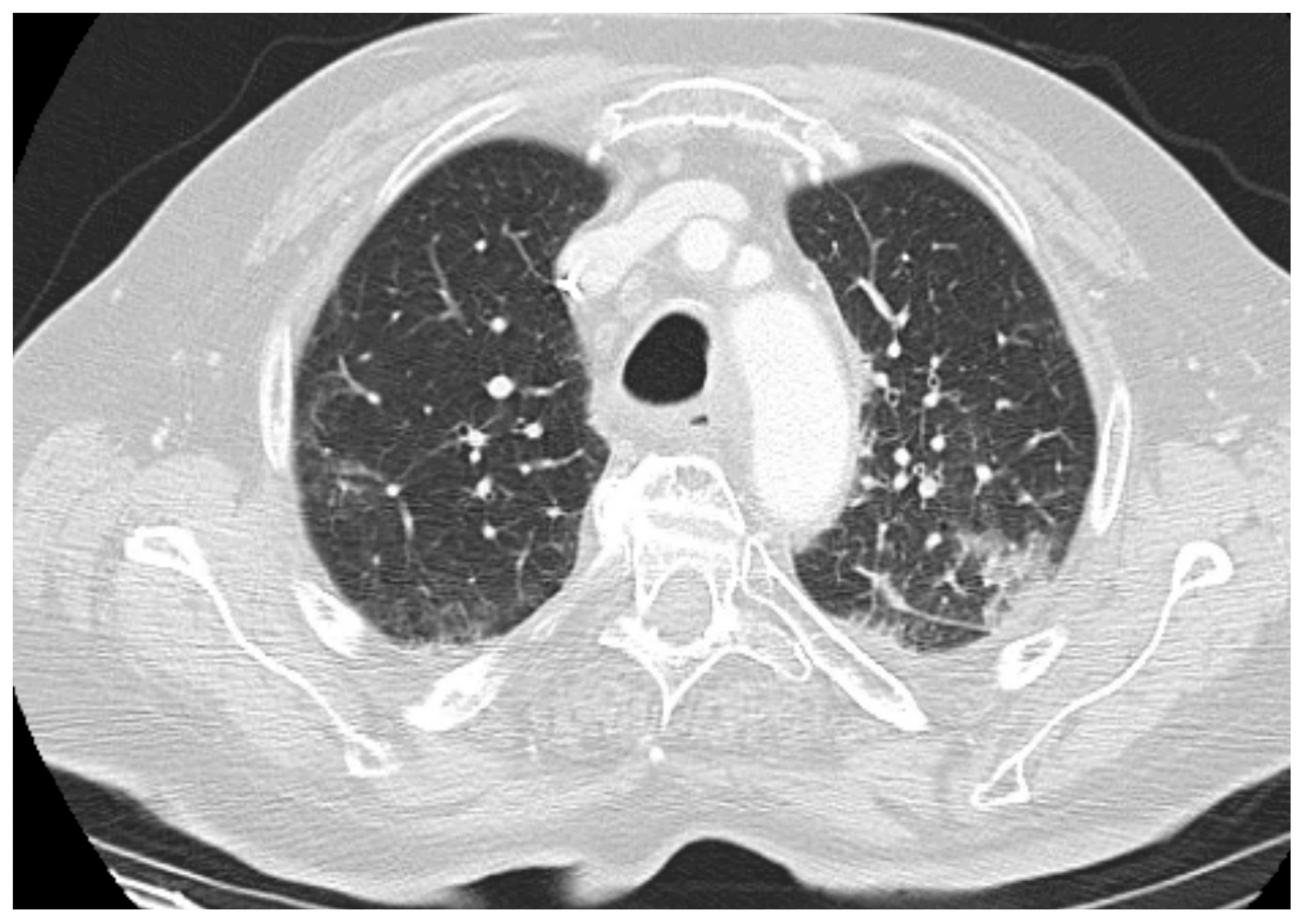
Contrast-enhanced CT scan of the chest demonstrating multiple tree-in-bud opacities and peribronchial consolidations
